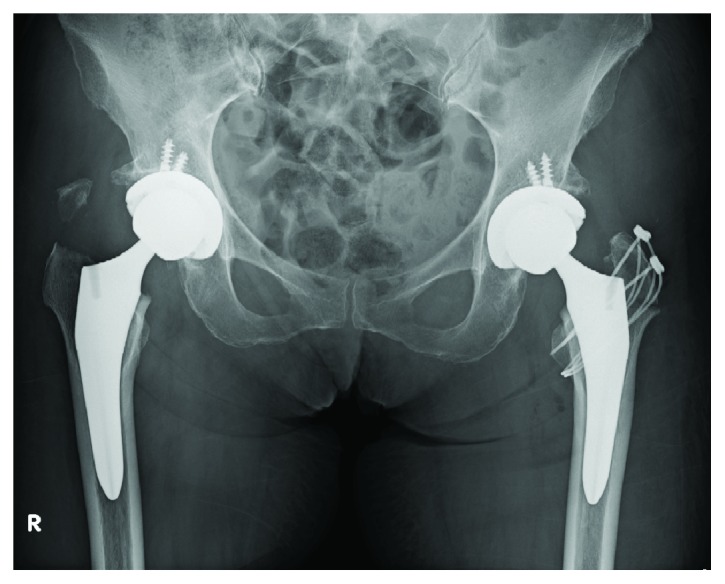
On the 20th postoperative day, right coxalgia emerged during a transfer exercise. The greater trochanteric avulsion fracture on the right side was detected on plain radiographs.
